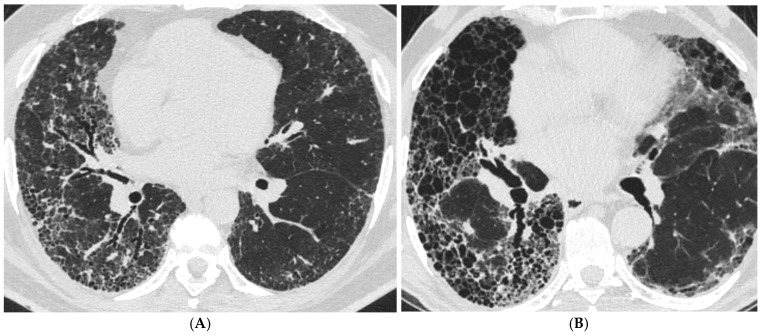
Axial CT scans of fHP with UIP-like HRCT pattern (A) and IPF/UIP (B). Extensive reticulation, traction bronchiectasis, air-filled cysts (probably honeycombing), and mild GGO are seen in both scans.
